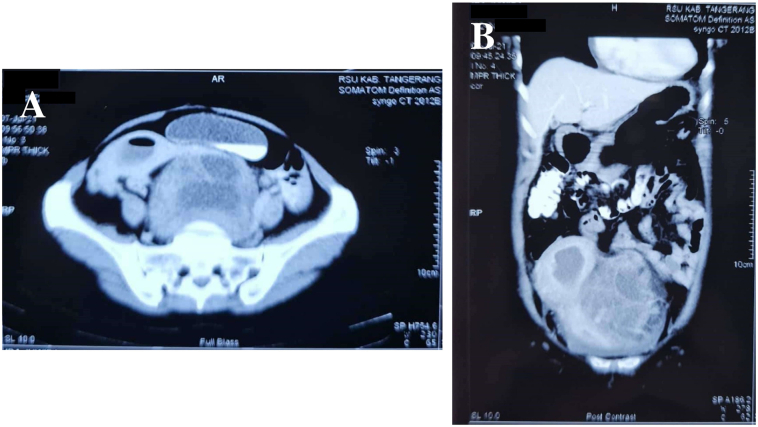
Abdominal CT scan of the patient in (A) axial and (B) coronal view showing multilocular cystic mass with thick septa and solid component intra lesion with enhancement pushing the uterus.
